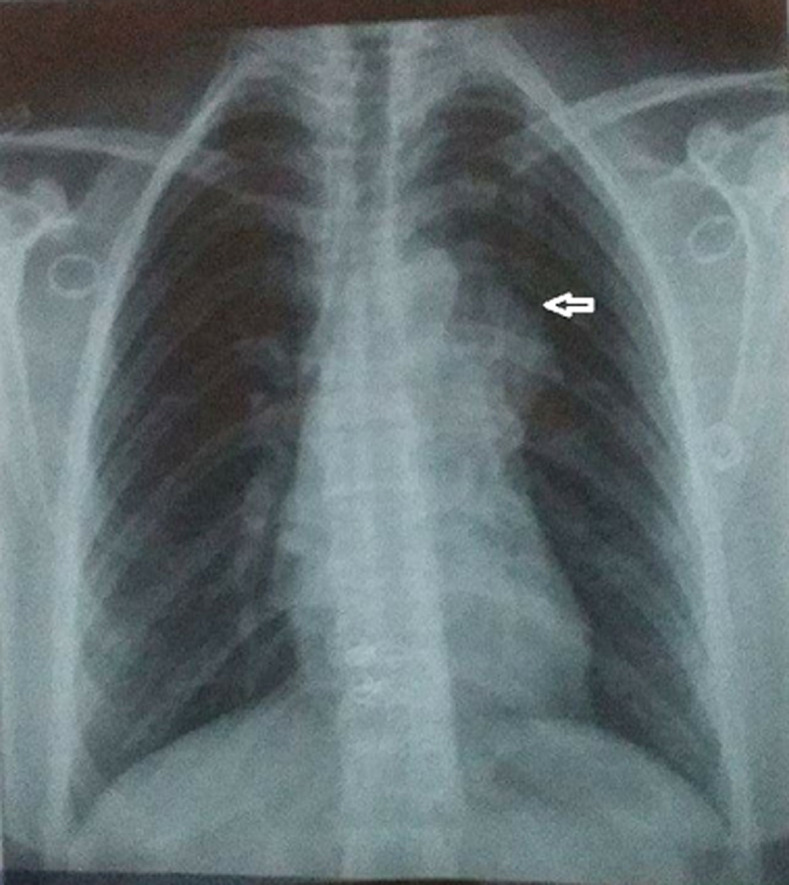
preoperative chest X-ray; left paracardiac opacity (arrow)
